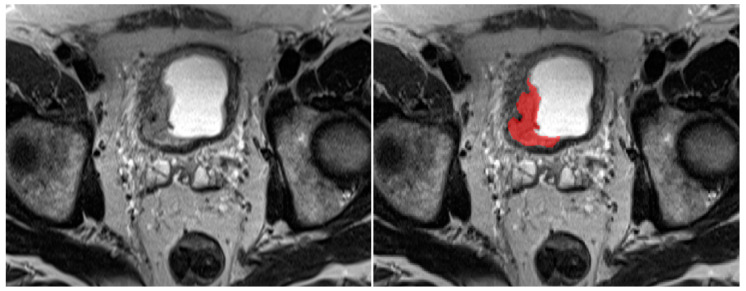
Axial T2-weighted image of a patient with bladder cancer and manual contouring of the tumor (red) on the right.
